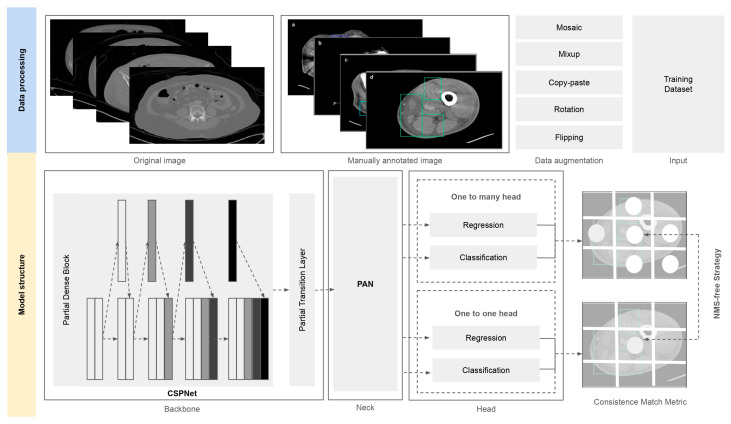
Overview of the YOLOv10-based detection framework for NSTI features on CT images. The model integrates a CSPNet backbone, PAN neck, and decoupled head with an NMS-free strategy.
